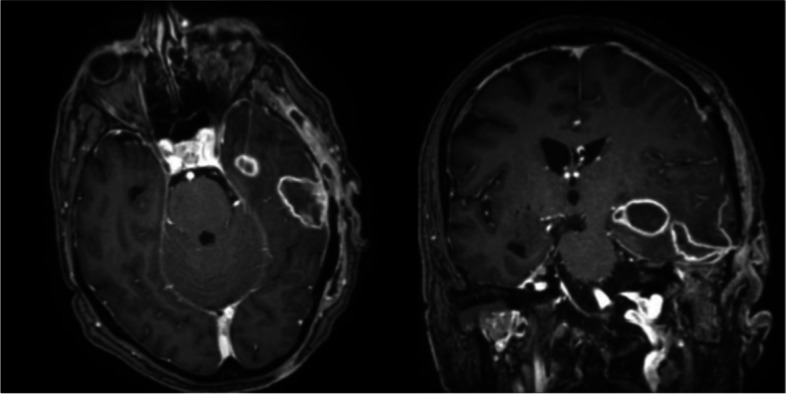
T1-weighted MR scans of brain, 5 weeks following neurosurgery
